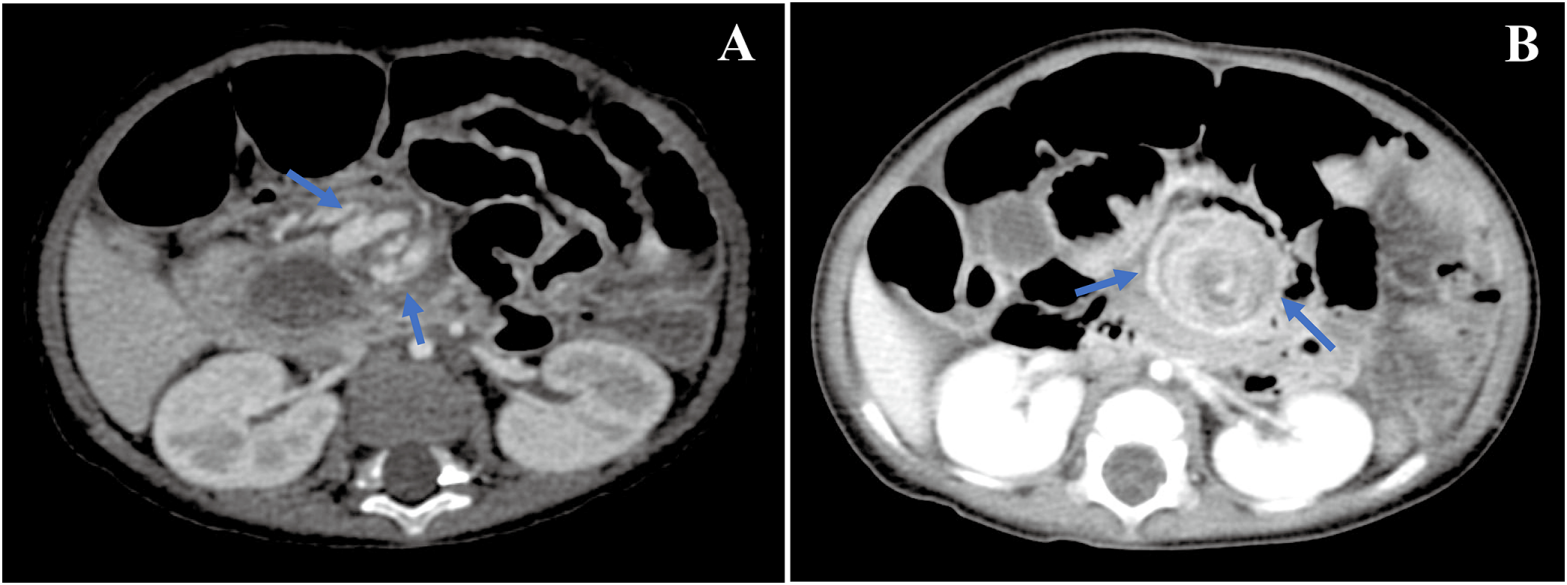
CT images. Whirlpool sign in the superior mesenteric vessel's branches was detected by an abdominal CT examination [(A) in case 1 and (B) in case 2].
